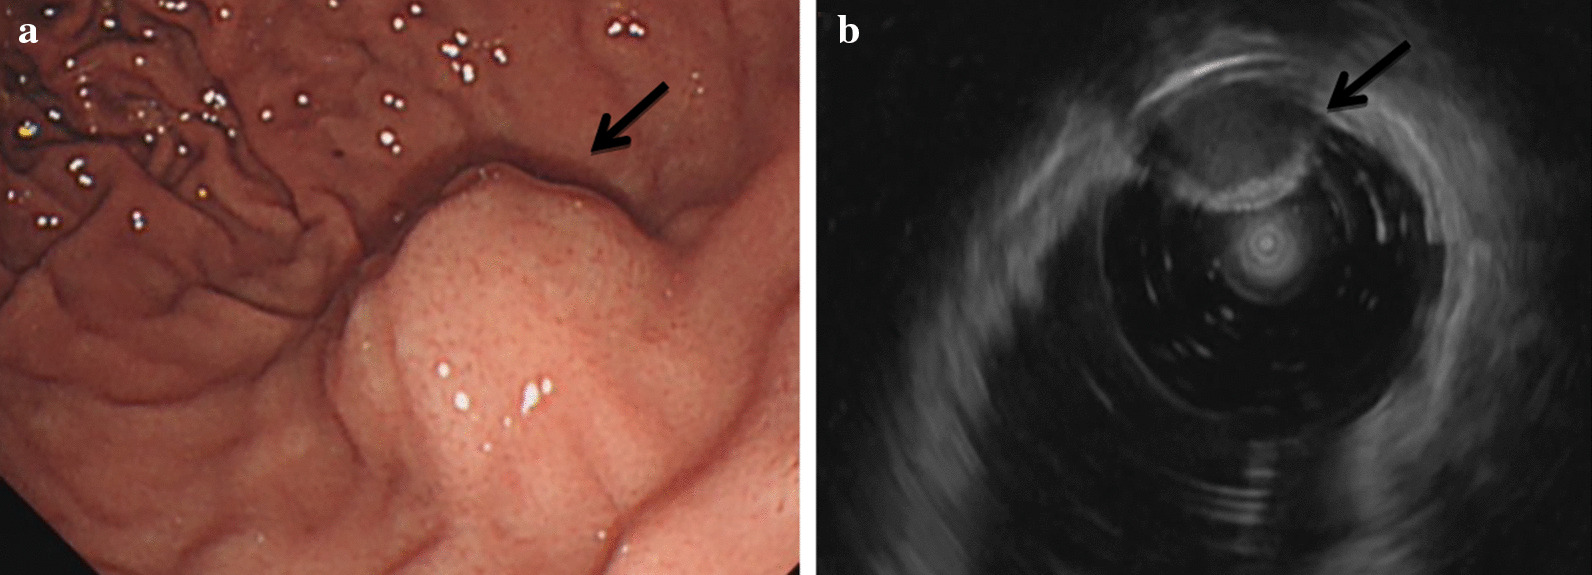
A 19-year-old male patient with stomach bleeding after drinking alcohol in 2020. Gastroscopy revealed a submucosal elevated lesion in the gastric fundus. Endoscopic ultrasonography revealed a tumor with low homogenous echogenicity originating in the gastric muscular layer. a Gastroscopy; b endoscopic ultrasonography
